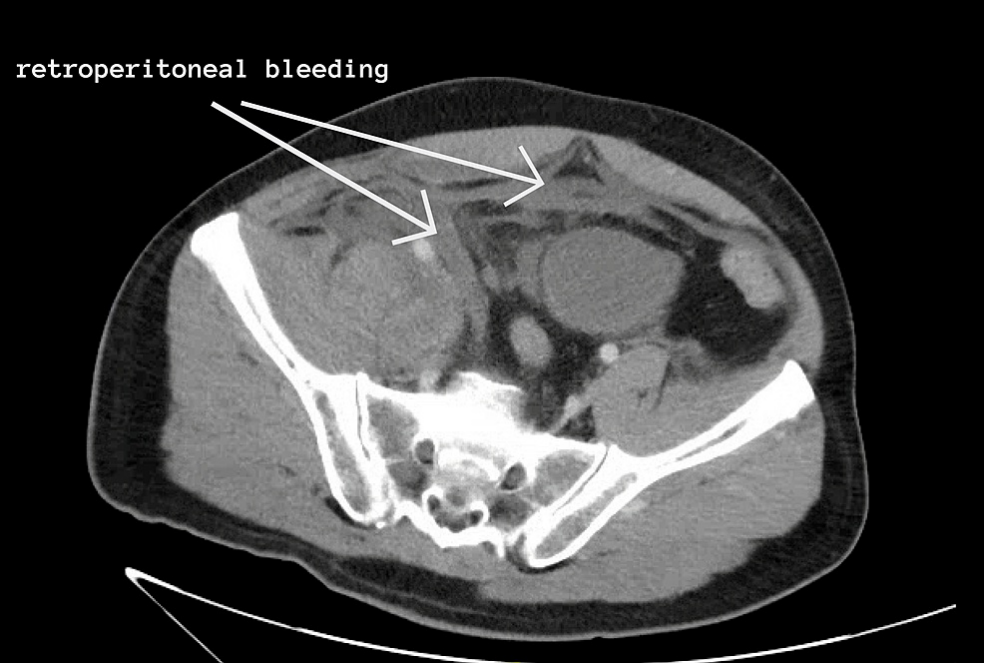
Abdominal CT with IV contrast showing acute hemorrhage in the inguinal and pelvic regions extending to the right psoas muscle and retroperitoneum.
